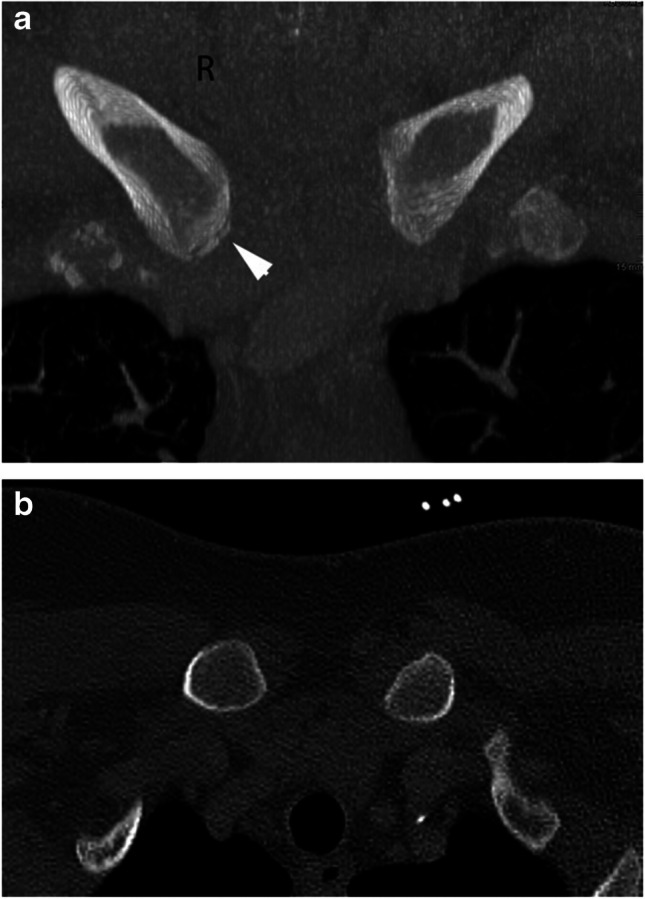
a/b Male subject aged 20 years and 10 months. The stages of maturity of the medial clavicles were 3c on the right and 4 on the left (CT MIP projection). He is the only study participant who reached maturity stage 4 before the age of 21. a Coronal plane. b Axial plane
